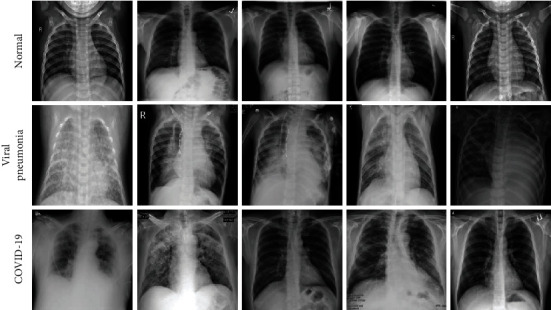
Some examples of chest X-ray images of three categories: normal (healthy person), viral pneumonia (patients suffering from viral pneumonia), and COVID-19 (pneumonia caused by COVID-19).
